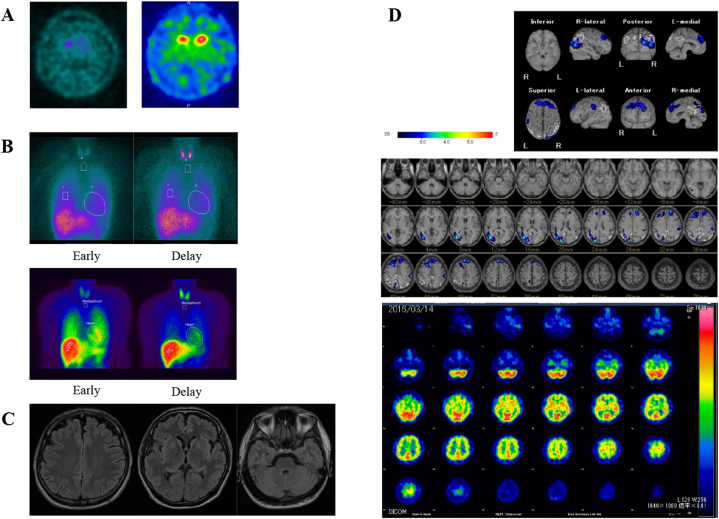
Neuroimaging of patients with*PARK7*variants. A: DAT-SPECT of Patients A–III–1 (left) and C-II-1 (right). B: ^123^I-MIBG myocardial scintigraphy of Patients A–III–1 (upper) and C-II-1 (lower). C: Brain MRI of Patient C-II-1. D: ^99m^Tc-ECD-SPECT of Patient A–III–1.
